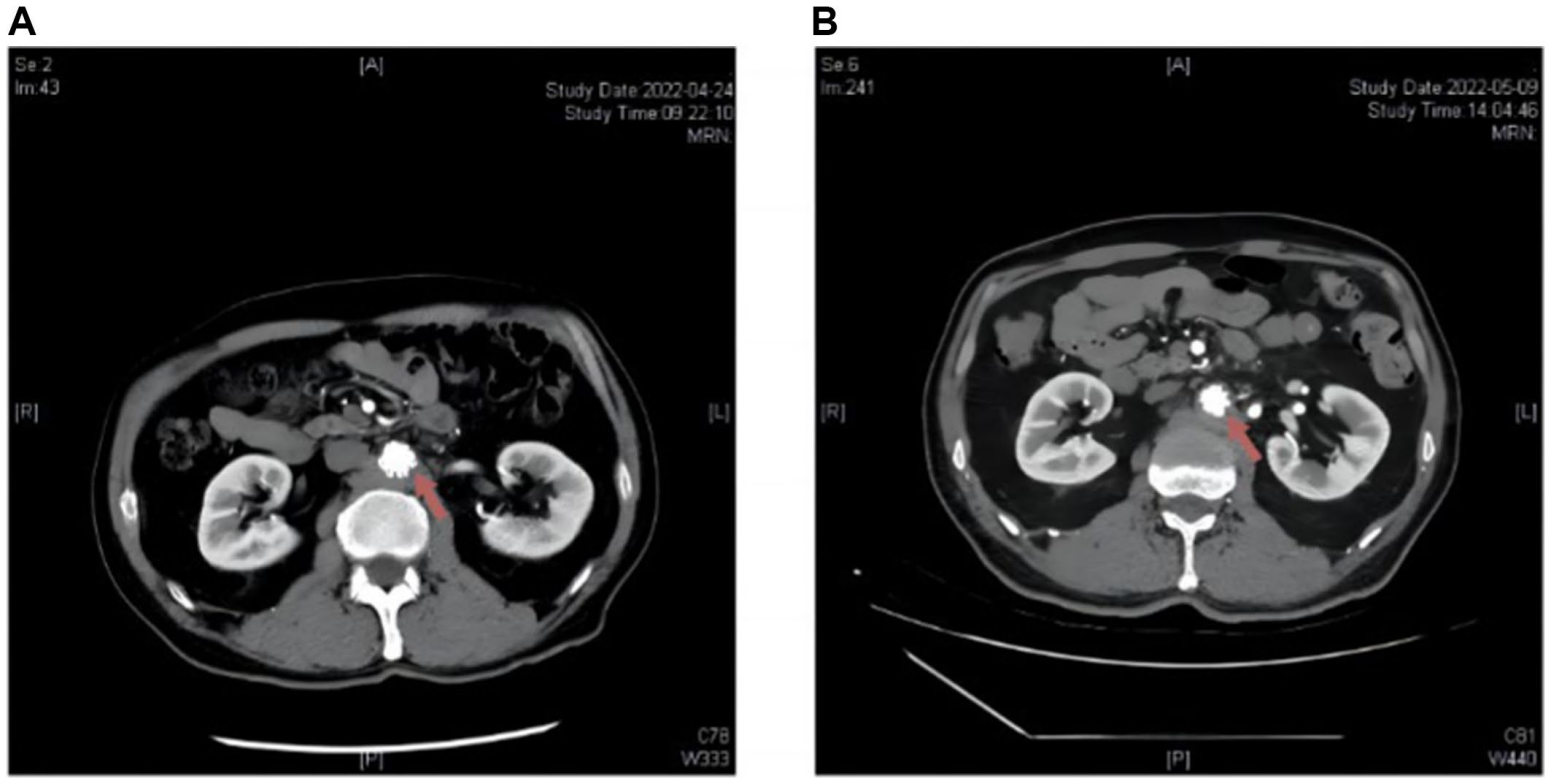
Radiological alterations on abdominal aortic CTA before and subsequent to antibiotic administration in Patient 2 post endovascular aneurysm repair (EVAR). (A) Upon admission: multiple patchy and nodular abnormal density shadows around the abdominal aorta. (B) After treatment: the abnormal density shadows around the abdominal aorta decreased compared to the previous findings.
